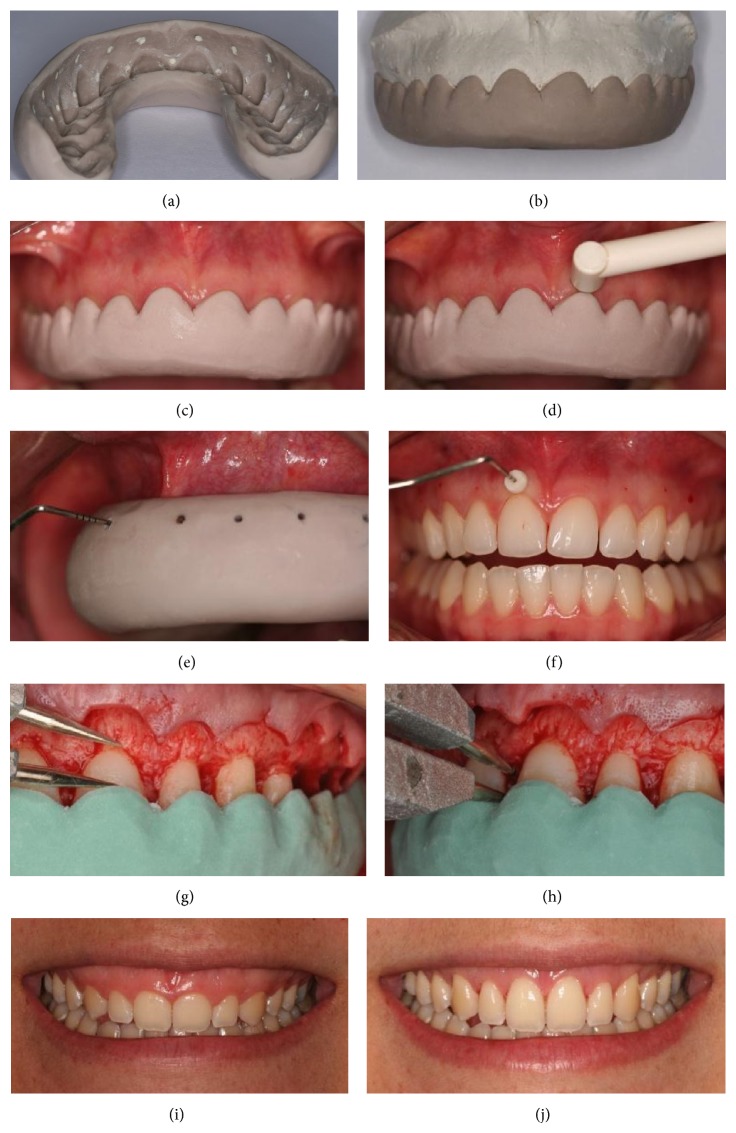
(a) Tomographic guide showing the marks made at the tip of each interproximal papilla and 3.0 mm above the gingival margin of each tooth. (b) Clinical guide following the contours of the gingival margin and the tips of the interproximal papillae. (c) Positioning the clinical guide to measure gingival thickness (GT) using ultrasound (US). (d) Positioning the US to measure GT. (e) Marking the point to measure GT. (f) Silicon limiter in contact with the external surface of the gum to measure GT. (g) Measuring the distance between the gingival margin and the vestibular bone crest (GMBC-V) at the highest point from the clinical guide margin to the vestibular bone crest. (h) Measuring the distance between the gingival margin and the interproximal bone crest (GMBC-I) from the point in the clinical guide which represents the tip of the interproximal papilla to the interproximal bone crest. (i) Patient's gummy smile. (j) Patient's smile after surgery.
